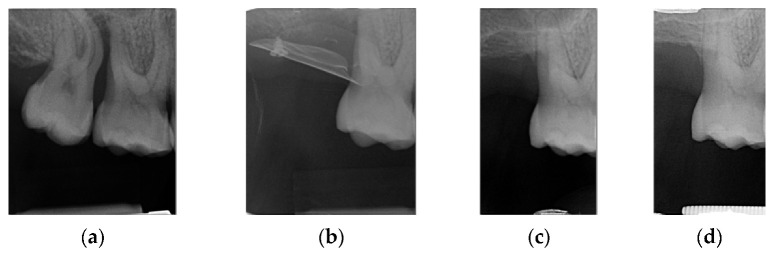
(a) Initial periapical radiograph; (b) periapical radiograph after foil placement; (c) periapical radio-graph after 6 months; (d) periapical radiograph after one year.
